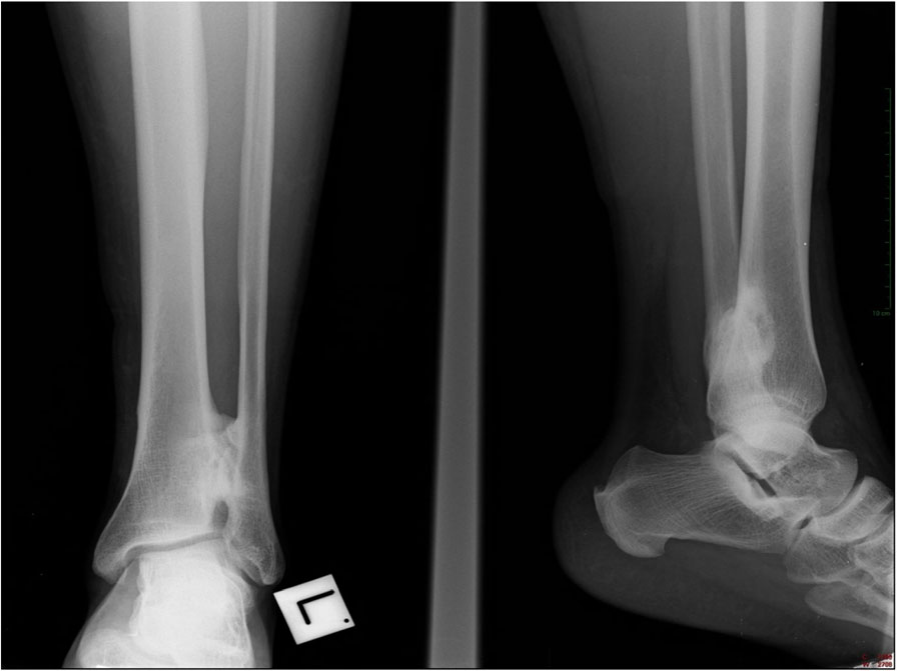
An antero-posterior and lateral radiographic view of our patient’s left ankle, taken 20 weeks after injury. Radio-opaque appearance is evident on the distal tibiofibular area, suggestive of tibiofibular synostosis formation
